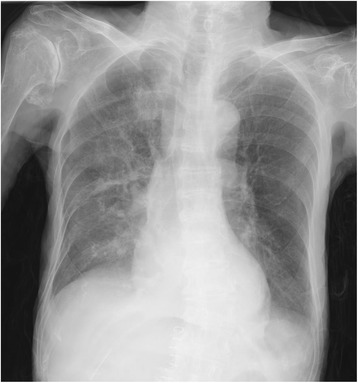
Radiographic image of the adult Bochdalek hernia after operation. Chest X-ray showed no recurrence
